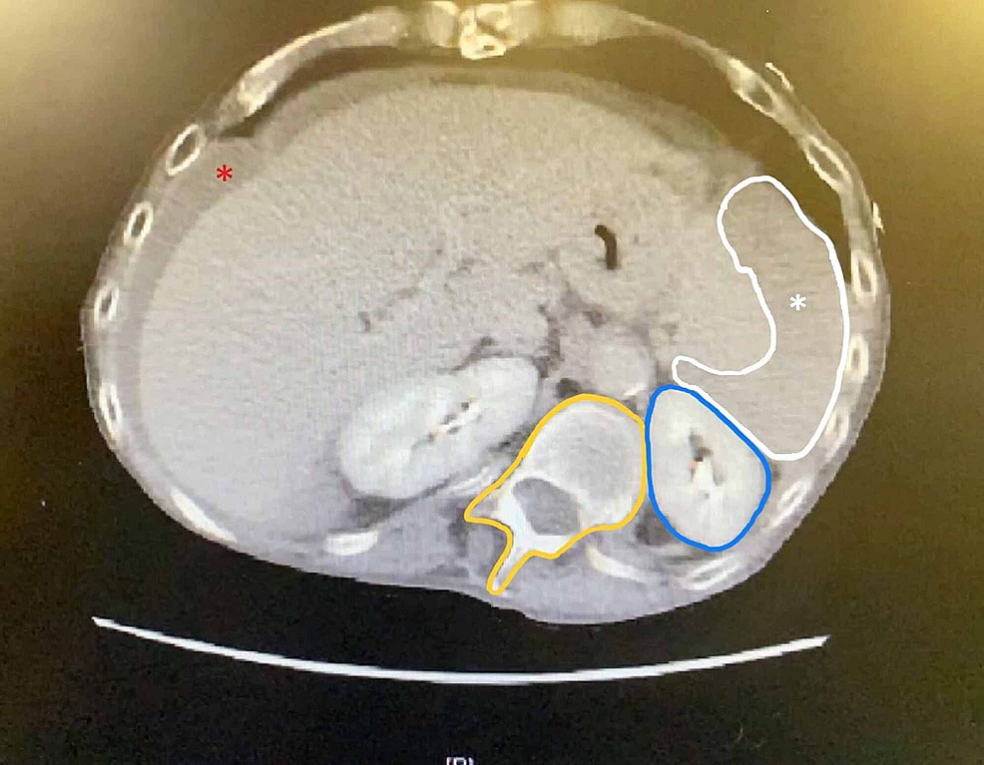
Axial view CT image. Extensive splenic hematoma (white asterisk and outline), hemoperitoneum (red asterisk), and scoliotic vertebrae (yellow outline) abutting the left kidney (blue outline) and injured spleen. CT, computed tomography
